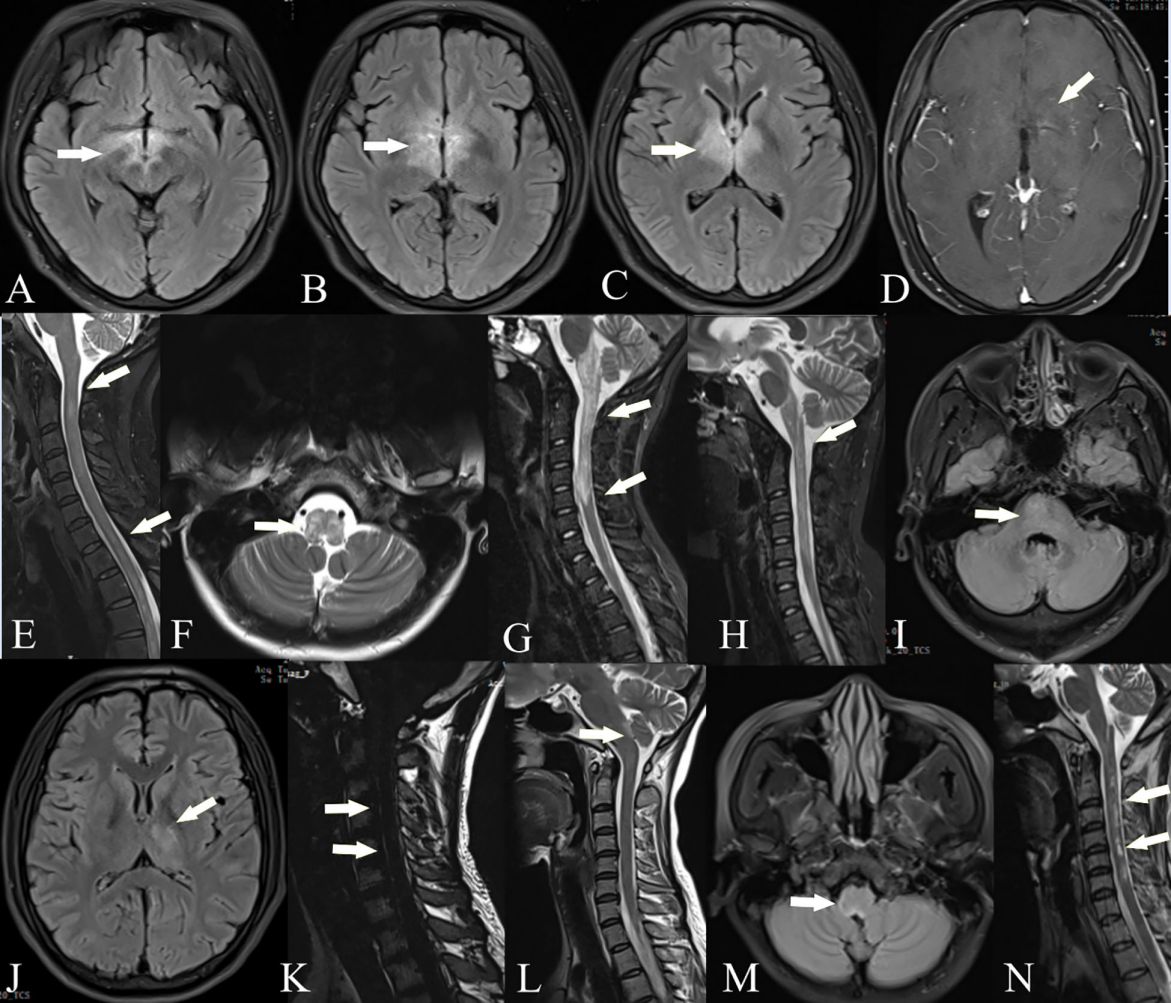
Brain and spinal cord MRI of GFAP-AQP4 overlapping syndrome. Patient 1: T2-FLAIR imaging showed lesions in the thalamus, fornix, optic chiasm, and around the third ventricle (A–C). Patient 2: Contrast-enhanced T1-weighted imaging showed spotty enhancement in the corpus callosum and around the third ventricle (D). Patient 3: T2-weighted imaging showed lesions in the spinal cord (E). Patient 5: T2-weighted imaging showed lesions in the medulla oblongata (F) and spinal cord (G); after treatment, the lesions were significantly reduced (H). Patient 6: T2-FLAIR imaging showed lesions in the paraventricular region, thalamus, and pons (I, J), with leptomeningeal enhancement in the cervical cord (K). Patient 7: T2-FLAIR imaging showed lesions in the area postrema (L, M). Patient 8: T2-weighted imaging showed lesions in the spinal cord (N).
